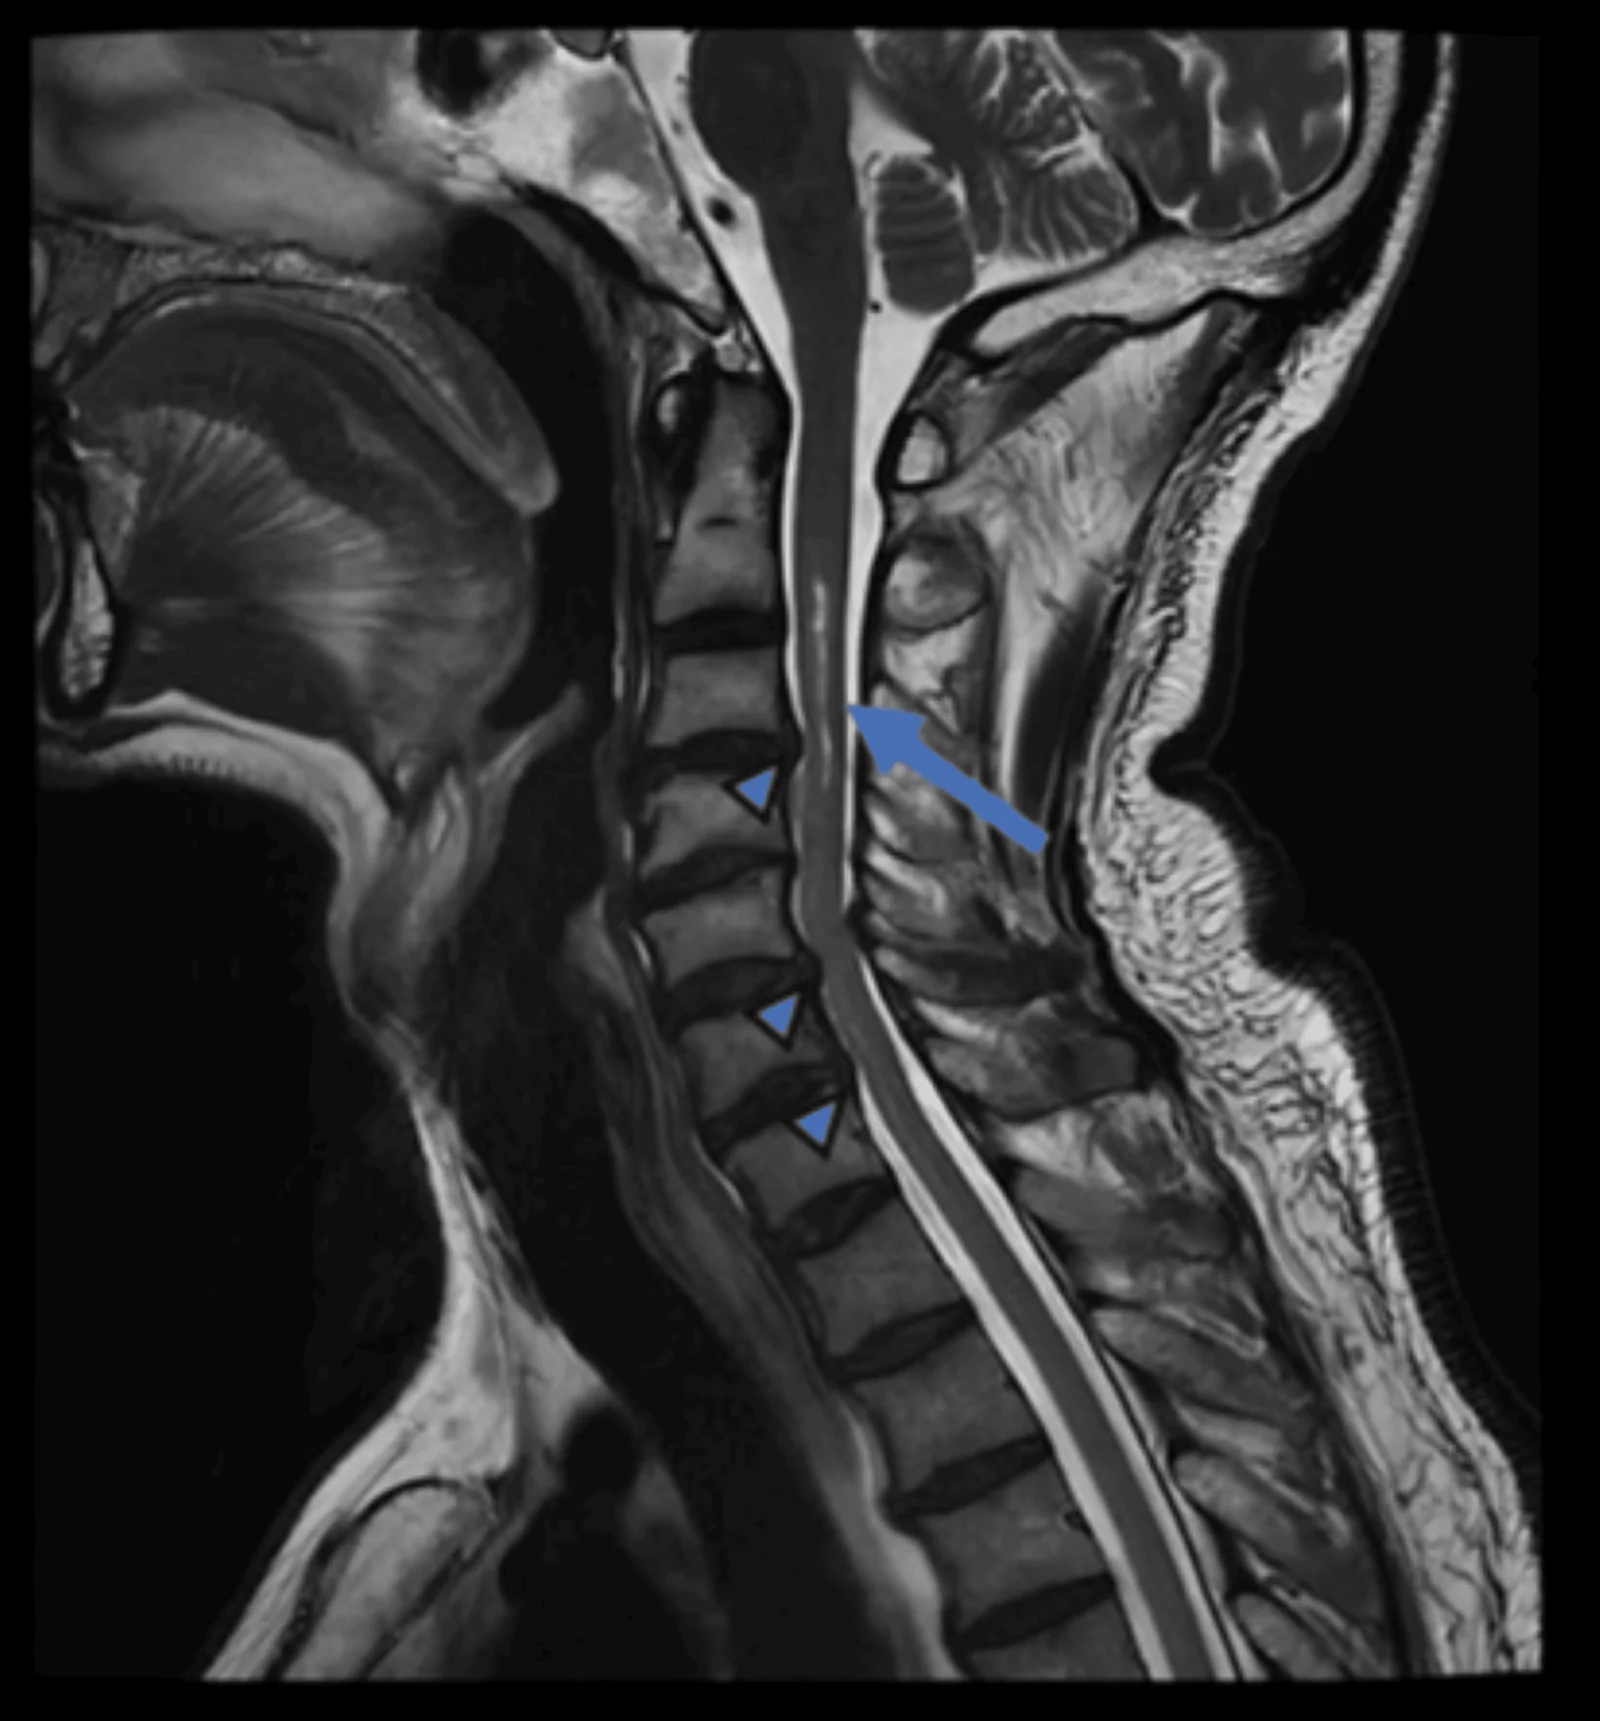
Sagittal T2-weighted MRI of the cervical spine Sagittal T2-weighted MRI of the cervical spine showing multilevel spinal cord compression from C3 to C7 (arrowheads). Central intramedullary T2 hyperintensity, extending from C2 to C5 (arrow). MRI: magnetic resonance imaging
